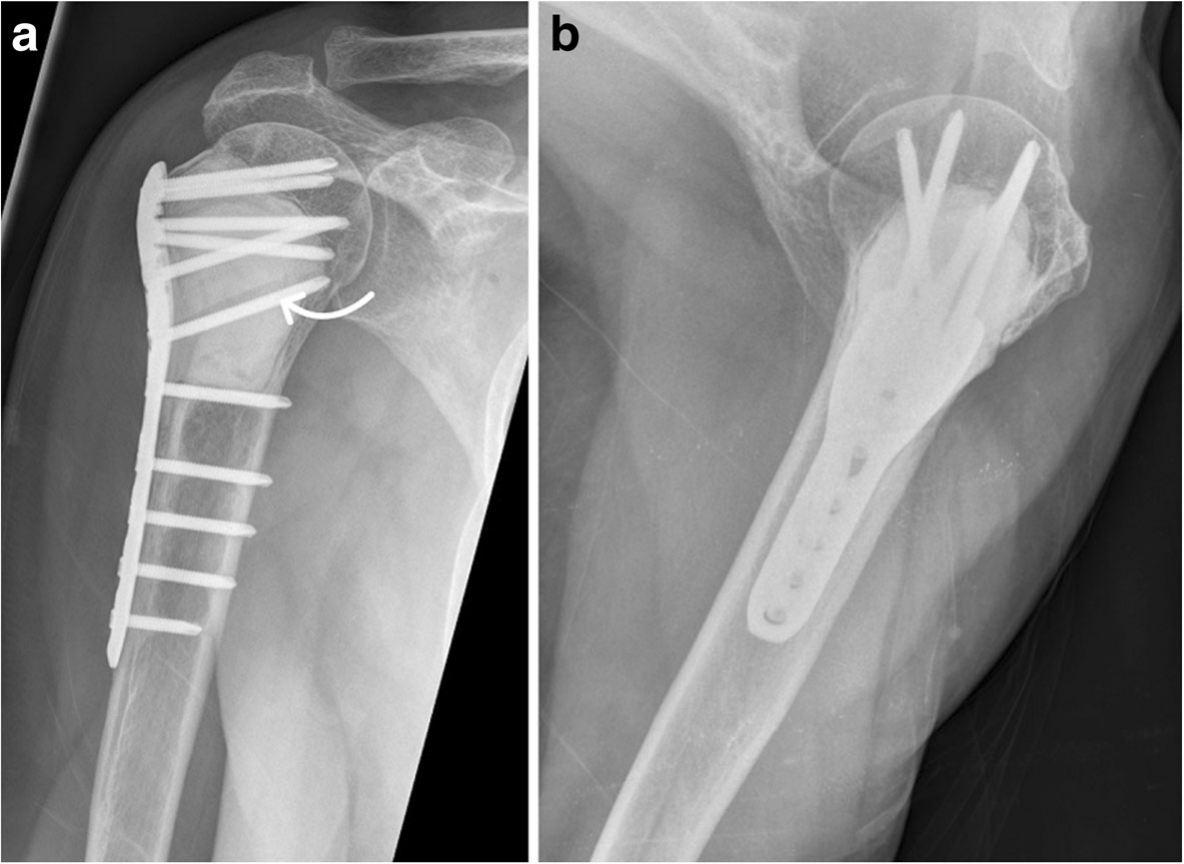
Bone cement filling with additional compound plate osteosynthesis after rigorous intralesional tumor resection. Plain radiographs in ap (a) and axial (b) view show additional stabilization with a proximal locking compression plate of the humerus with integration of the screws inside the bone cement (arrow) to potentially increase stability of the proximal humerus
